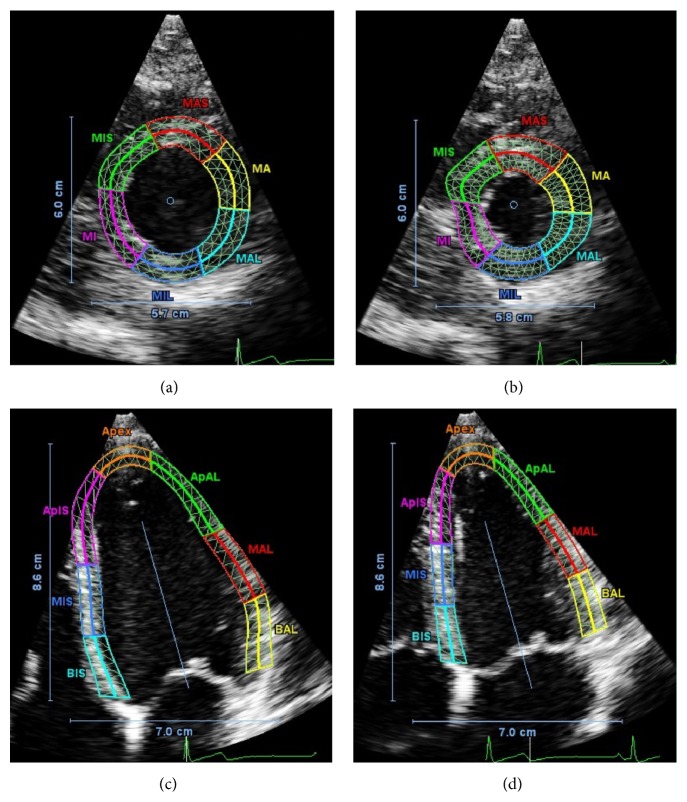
Example images (short axis (a, b) and long axis (c, d)) from echocardiography of a single control patient. SA images are at the midlevel plane, as viewed from the apex of the heart. LA images are in the four-chamber plane (AP4). The colored overlays indicate the myocardial regions considered in the estimation of regional LV strain and rotation as defined in Figure 1. The labels on each region indicate the subdivision in both longitudinal and circumferential directions. Preceding a region, “A” represents apex, “M” represents mid, and “B” represents base. For example, “MAL” represents mid plane, anterior lateral region. Each region is further subdivided into the epicardial and endocardial regions by the bold solid line in the center of each colored region.
